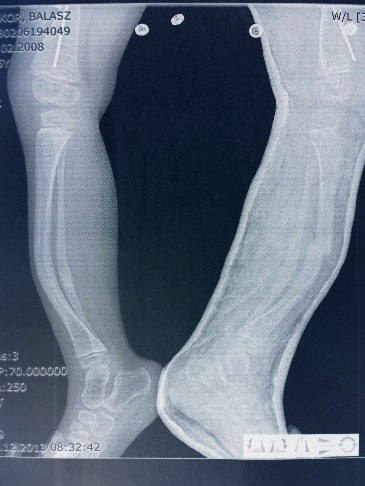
X-ray showing severe bowing of both tibias (approx. 300). Left tibia has a displaced diaphyseal fracture produced while walking
